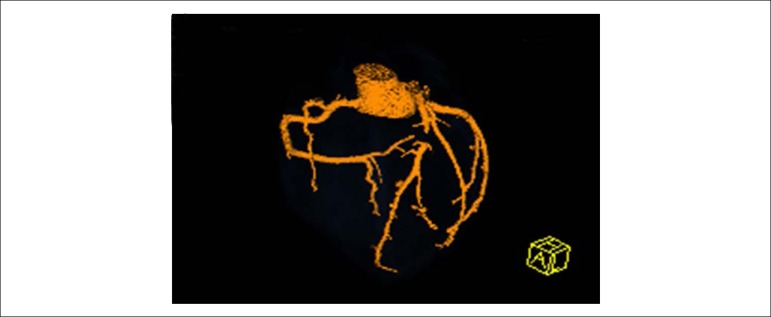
CT angiography: proximal obstruction of the anterior descending artery that receives collateral circulation of the right coronary artery and the circumflex coronary artery.
